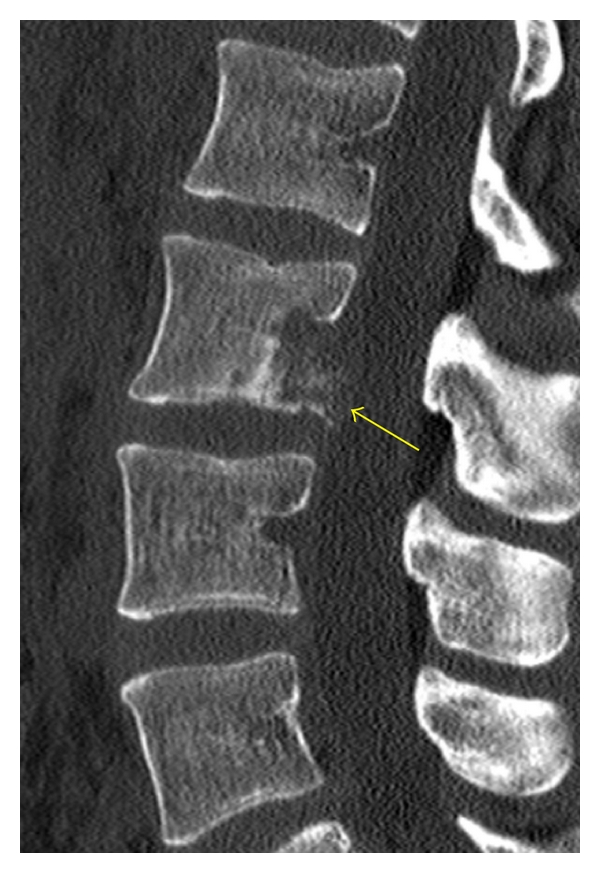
Sagittal CT reformation in bone algorithm depicts a cortical break in the posterior cortex of the L3 vertebral body (yellow arrow) due to a metastatic focus.
